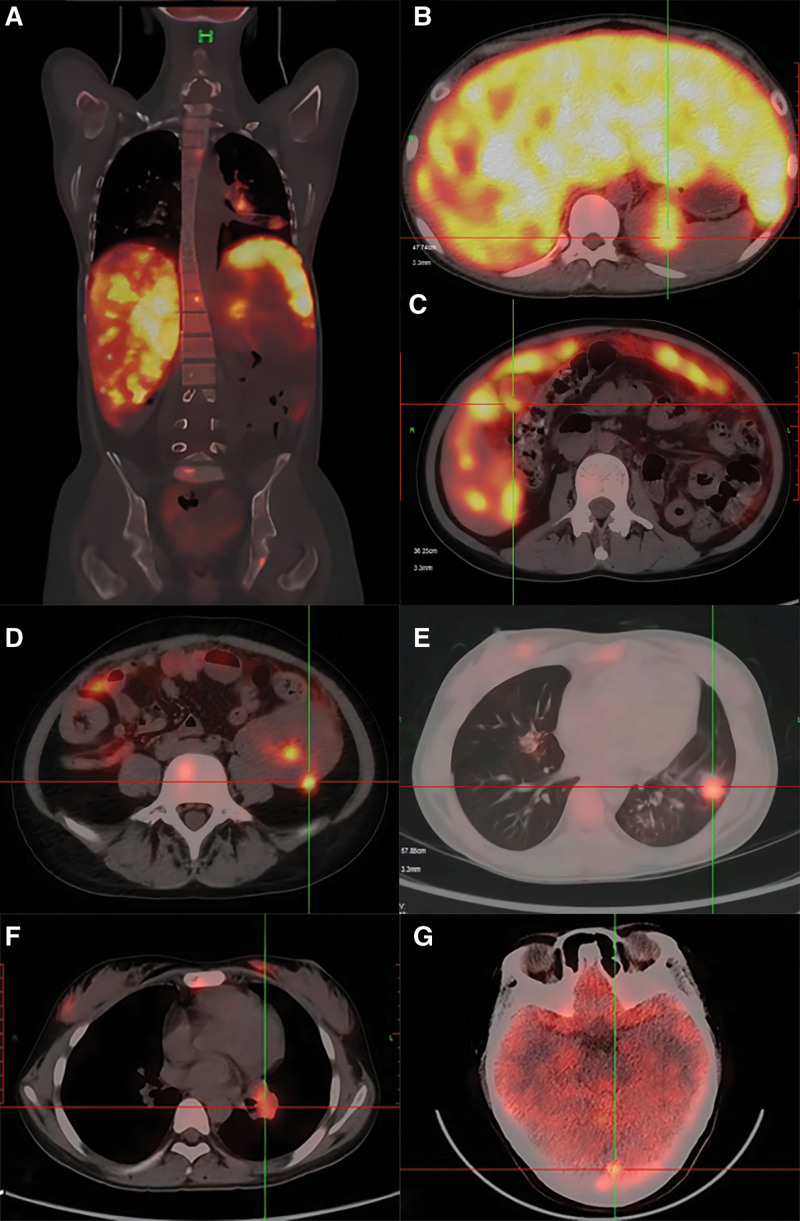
PET-CT images. (A) Showing multiple hypermetabolic lesions throughout the body. Increased and enlarged peritoneal and retroperitoneal lymph nodes with partially elevated metabolism; multiple elevated bone metabolism throughout the body (SUV_max_ 5.7). (B) Heterogeneously elevated metabolism in the liver (SUV_max_ 13.2), thickened gallbladder wall with elevated metabolism (SUV_max_ 8.3). (C) Locally elevated metabolism in the spleen (SUV_max_ 6.2) and splenic hilar nodule (25 × 15 mm). (D) Transplanted kidney localized metabolic increase (SUV_max_ 10). (E and F) left lower lung nodule (24 × 16 mm), metabolic increase (SUV_max_ 4.4), mediastinum and left hilar (SUV_max_ 6.1). (G) hypothalamic area, left occipital lobe metabolic increase (SUV_max_ 4.2), the above considered possible neoplastic lesions. SUV = standard uptake value, PET-CT = positron emission tomography-computed tomography.
